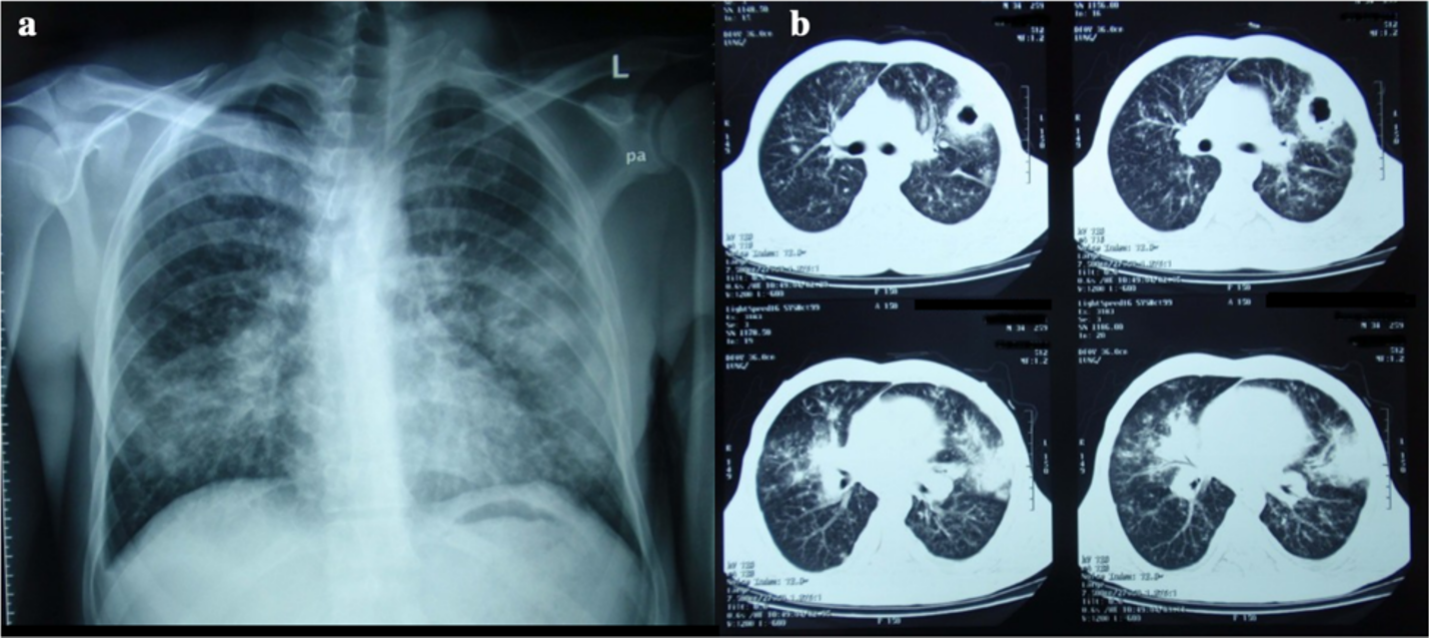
Chest X-ray (a) and contrast-enhanced computed tomography scan (b) of our patient at admission demonstrating bilateral infiltrates located mainly at the lower lung lobes and a cavitary lesion at the lingula.
